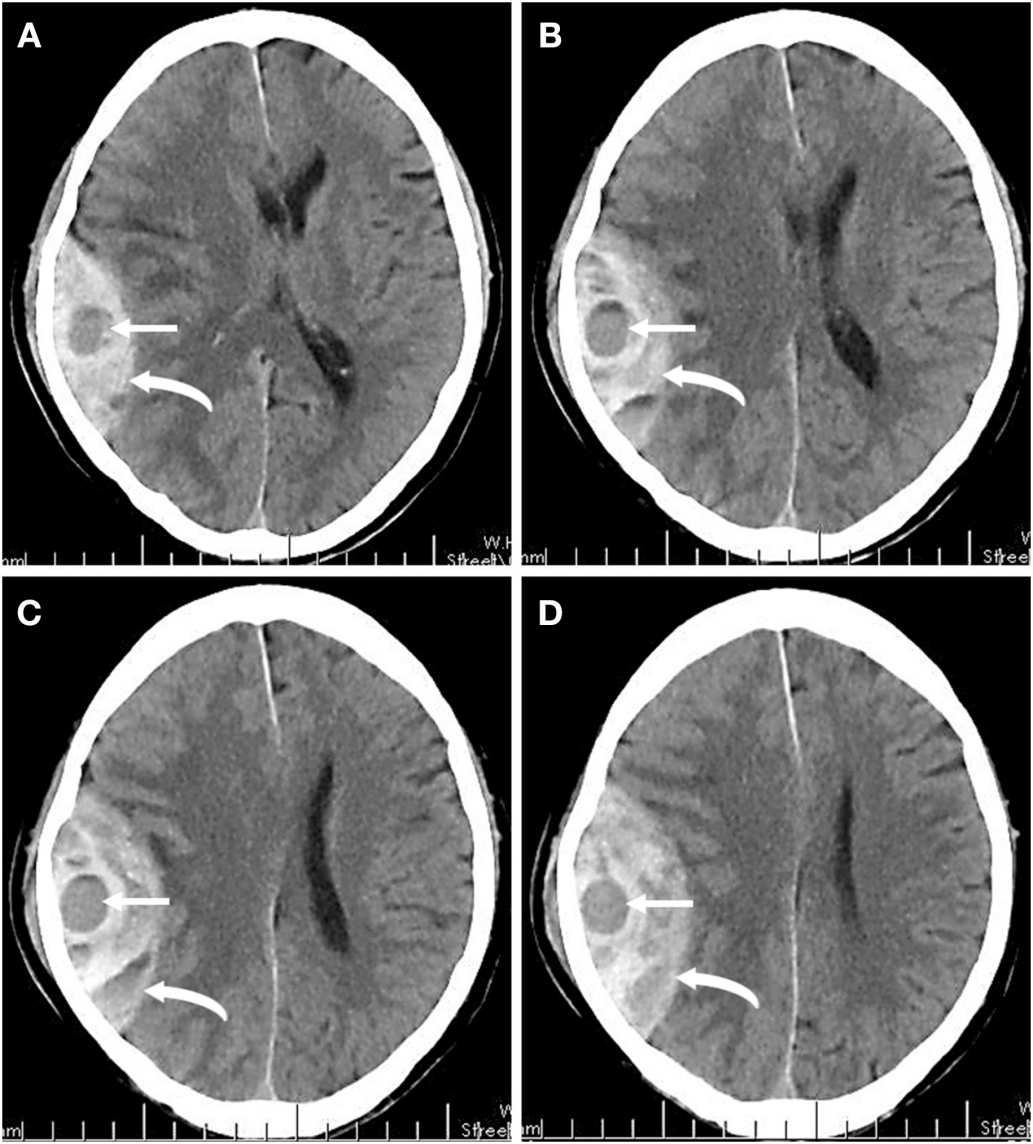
(A–D) A series of non-enhanced brain CT images of one patient at 2 h after head trauma. Right temporoparietal hyperattenuating epidural hematoma (curved arrows) with local hypoattenuating (straight arrows) represents the swirl sign.
